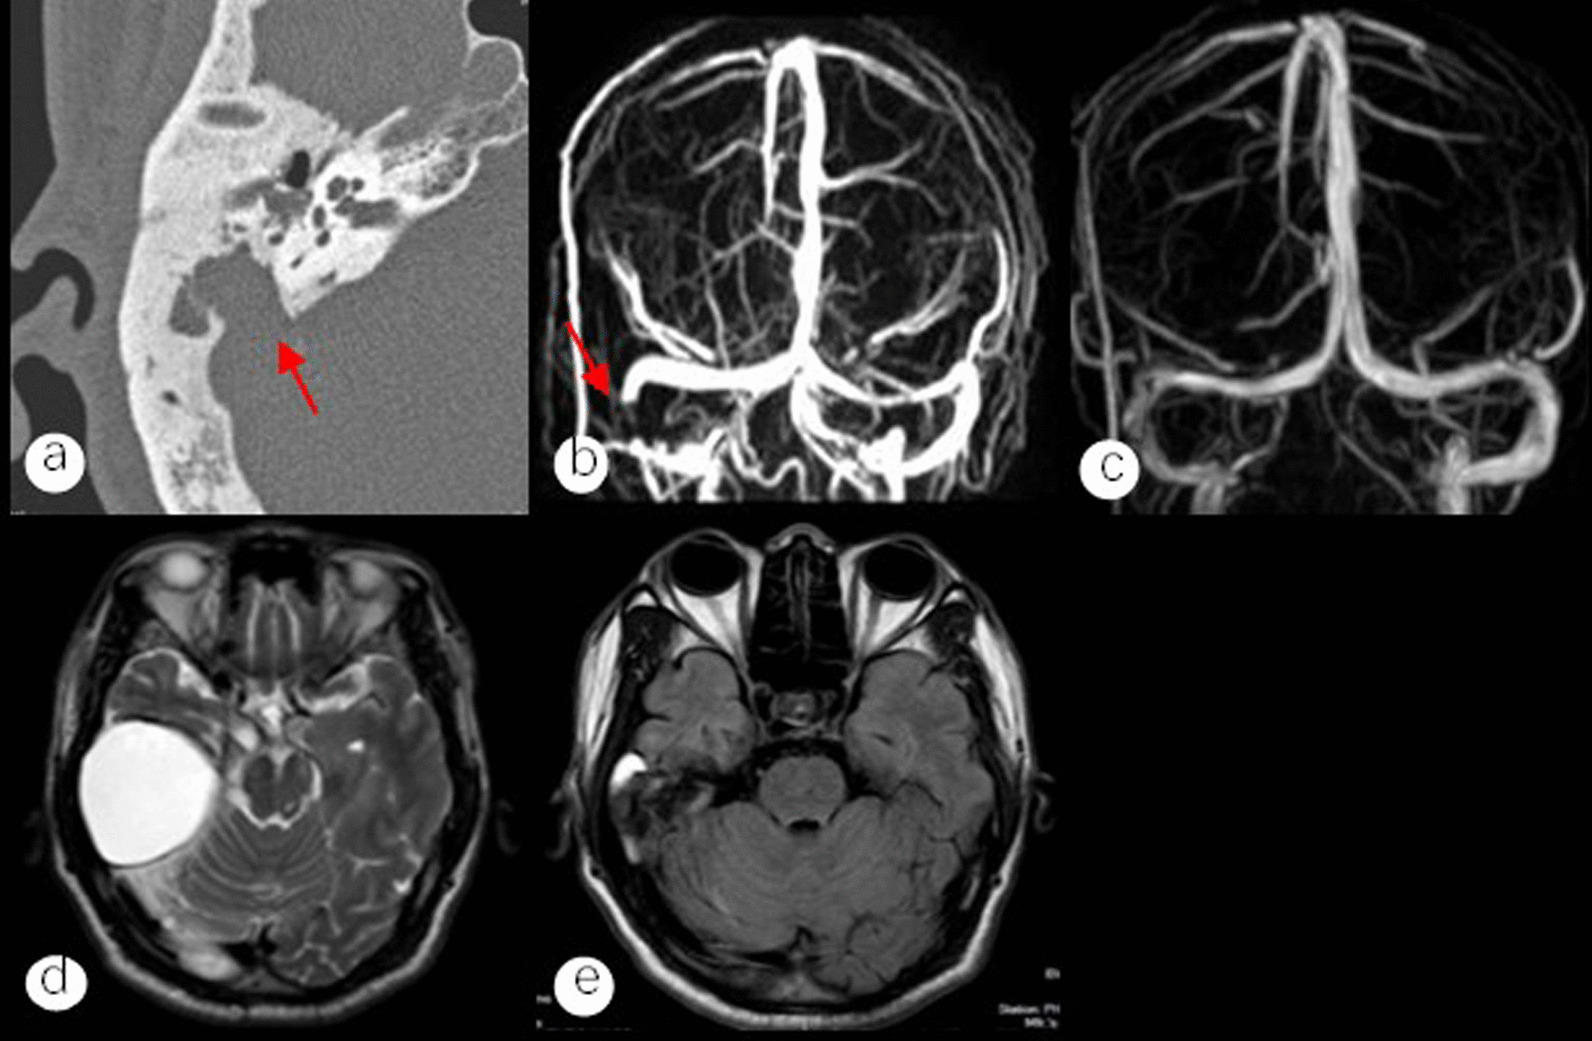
a Axial temporal bone CT with extensive bone destruction in the wall of the sigmoid sinus connected to the middle ear cavity (arrow); b Coronal MRV with no visualization of the right sigmoid sinus (arrow), confirming the diagnosis of sigmoid sinus thrombophlebitis; c MRV in sigmoid sinus thrombophlebitis patient 5 years after surgery suggests return of recanalization of right sigmoid sinus; d Axial MRI T1 image showing that this patient also had a combined temporal lobe brain abscess; e Axial MRI 3 months after tympanoplasty with significant resorption of the brain abscess lesion compared to the preoperative period
